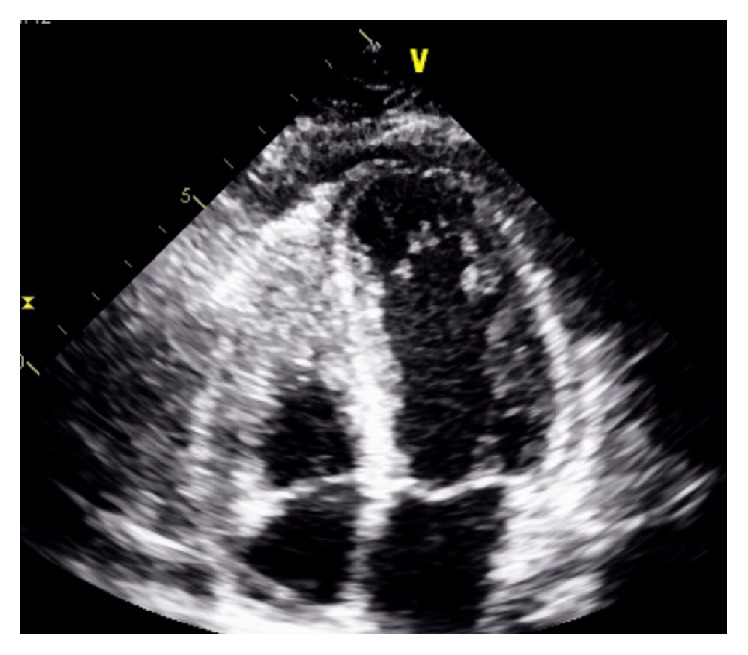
Transthoracic echocardiogram.
